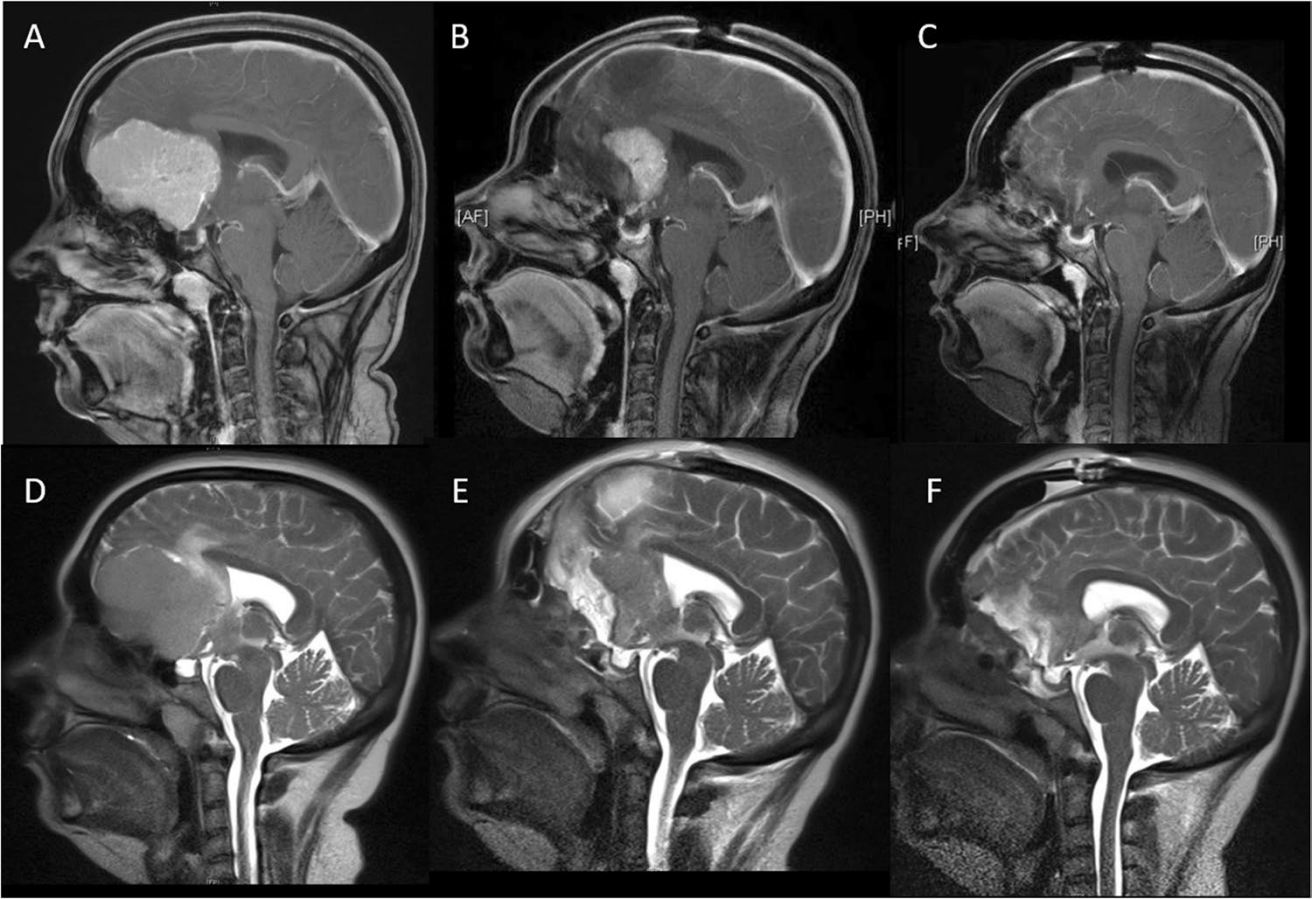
Sagittal MRI images T1-weighted gadolinium-enhanced and T2-weighted showing the perilesional oedema. A, D: Preoperative. B, E: One day after first operation showing the shell-shaped tumour remnant. C, F: One day after second operation with complete removal of the tumour
